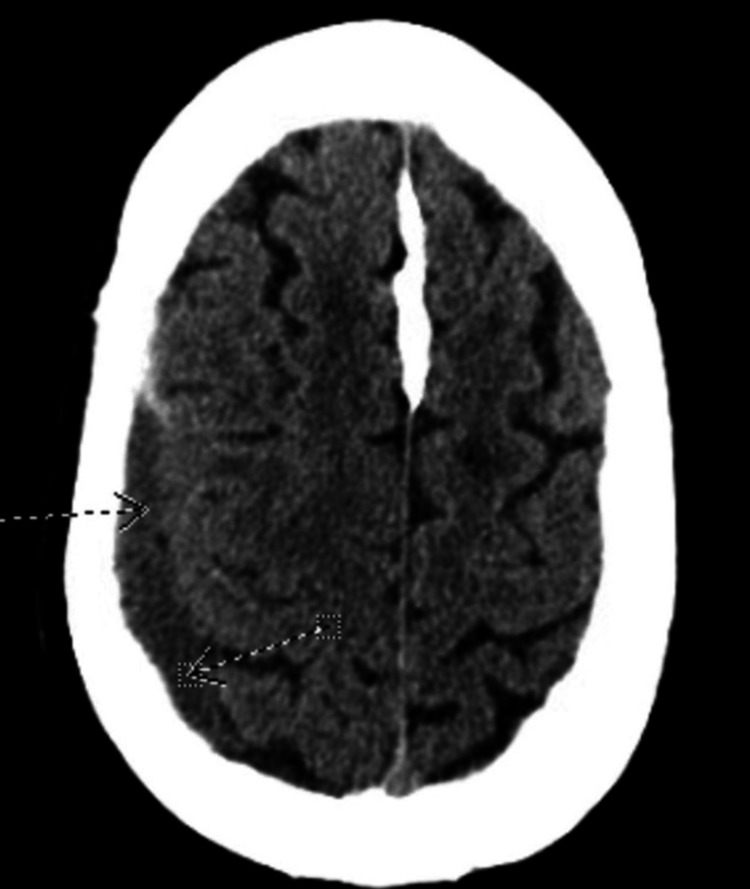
Computed tomography scan of the brain showing a right-sided hypodense collection (see arrows) in keeping with a chronic subdural hematoma
